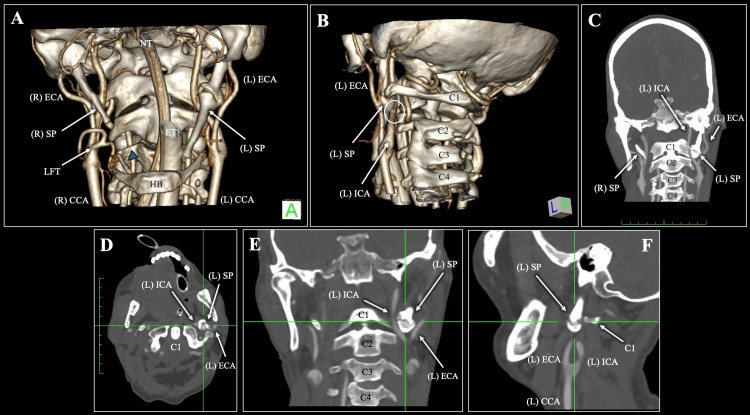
Three-dimensional reconstructions (A, B), maximum-intensity projection (C), and multiplanar reconstructions (D-F) of the patient’s multidetector computed tomography angiogram (CTA), demonstrating bilateral asymmetric ossification of Reichert’s cartilage. The blue arrowhead in (A) indicates the distal ossified segment of the right styloid process. (A) Anterior view. (B) Left lateral/posterior oblique view. (C) Coronal maximum intensity projection. (D) Axial reconstruction. (E) Coronal reconstruction. (F) Sagittal reconstruction. CCA: common carotid artery, ECA: external carotid artery, ICA: internal carotid artery, SP: styloid process, HB: hyoid bone, LFT: linguofacial trunk, ET: endotracheal tube, NT: nasogastric tube, L: left, R: right, C1: atlas, C2: axis.
